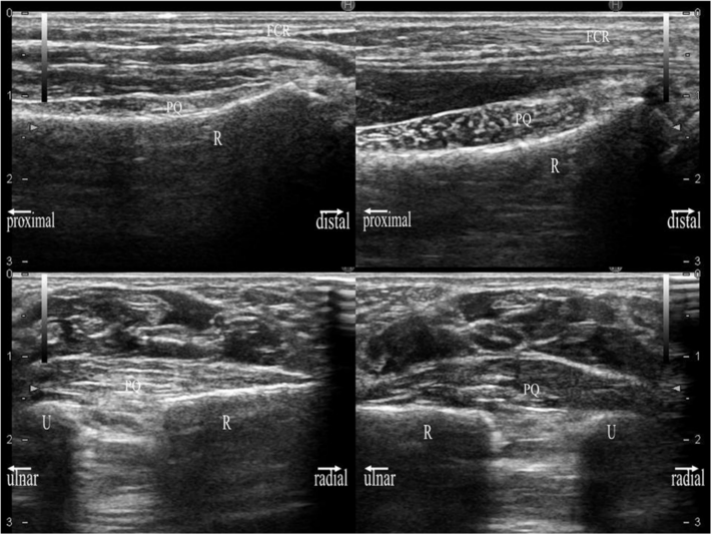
Sonographic images of a 59-year-old woman with a diagnosis of wrist joint effusion of the right hand. Sonographic images in the longitudinal (upper image) and transverse (lower image) planes were obtained using a 13.5-MHz transducer. Each image shows a side-by-side comparison of the right hand (right image) and left hand (left image). The PQ of the right hand is locally thickened. The PQ shows a convex appearance only in right hand.
